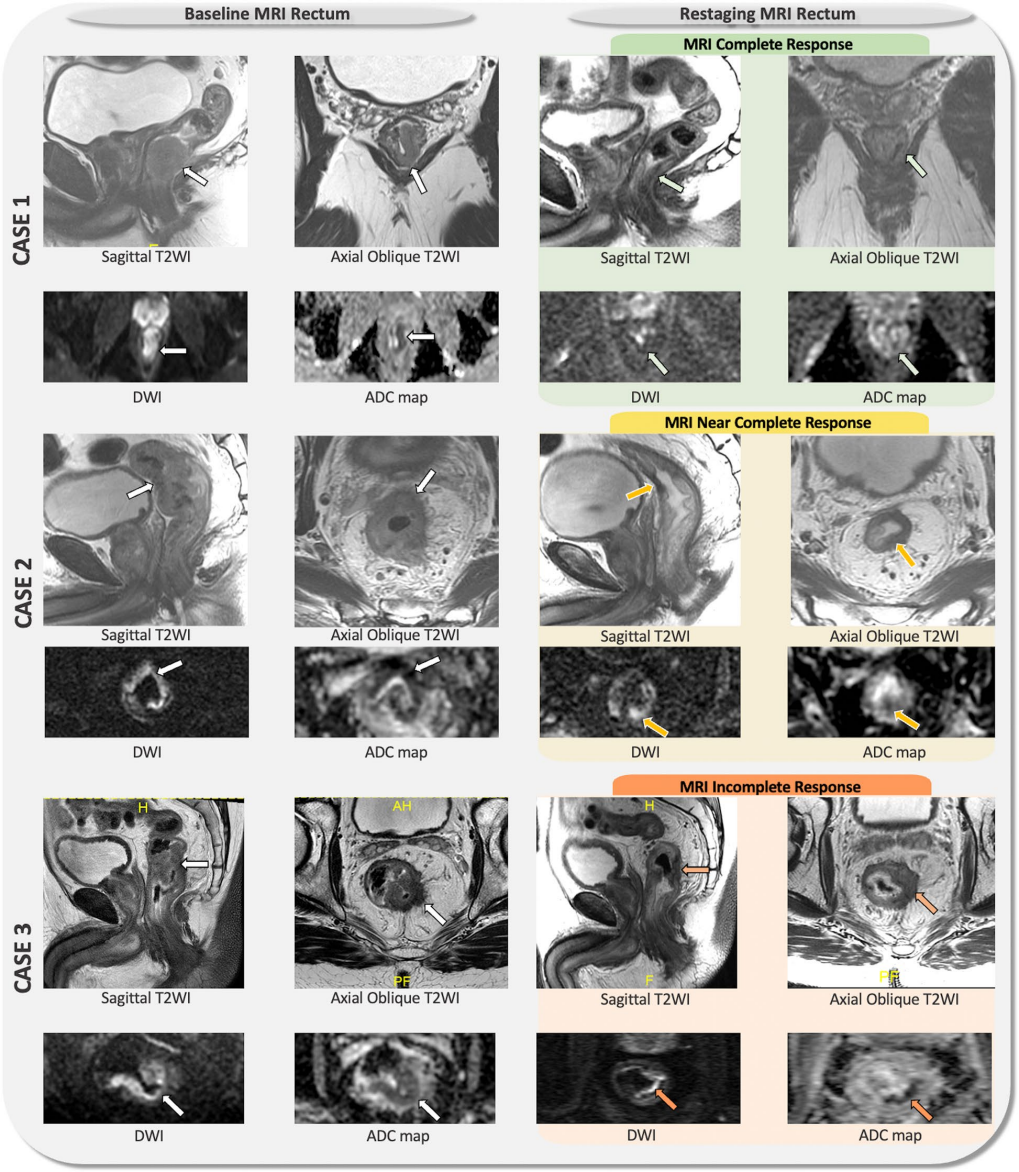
Baseline and re-staging MRI of the rectum of patients with complete response (case 1), near complete response (case 2) and incomplete response (case 3) based on the MRI assessment is shown. The white arrows on baseline MRI rectum indicate the primary rectal tumor, which shows intermediate signal intensity (SI) on T2-weighted imaging (T2WI), with high SI on diffusion-weighted imaging (DWI), and low SI on the ADC map, indicating restricted diffusion within the primary tumor.  Re-staging MRI on case 1 shows clinical complete response (green box) which is characterized by normalized rectal wall or only fibrosis (low signal intensity on T2WI, green arrows on T2WI) and no areas of restricted diffusion on DWI and ADC map (green arrows on DWI and ADC map).  Re-staging MRI on case 2 shows near complete response (yellow box) defined as small area of intermediate SI on T2WI within the tumor bed fibrosis (yellow arrows on T2WI) and small areas of restricted diffusion (yellow arrows on DWI and ADC map).  Re-staging MRI on case 3 shows incomplete response (orange box) characterized as significant areas of viable tumor with intermediate SI on T2WI within the tumor bed (orange arrows on T2WI) with restricted diffusion (orange arrows on DWI and ADC map). Reviewing the baseline MRI is highly recommended, as it helps to localize the tumor bed and guide the MRI to the appropriate angulation for high-resolution axial oblique T2WI acquisition perpendicular to the tumor bed
